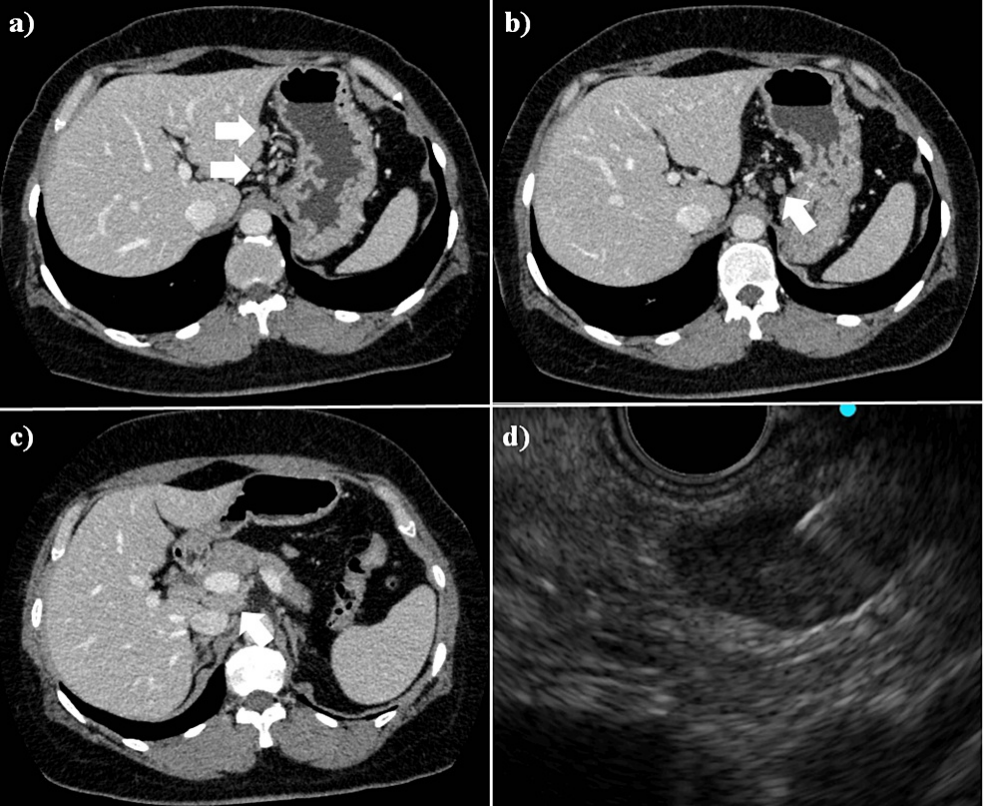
Computed tomography (CT) and endoscopic ultrasound (EUS) of the case 2 patient. Computed tomography (CT) shows the lymphadenopathy (arrows) affecting the (a and b) gastric regional lymph nodes and the c) hepatoduodenal ligament lymph node. d) Endoscopic ultrasound (EUS) shows lymphadenopathy as a hypoechoic mass lesion in the hepatoduodenal ligament. EUS-FNA of the lymphadenopathy is performed.
